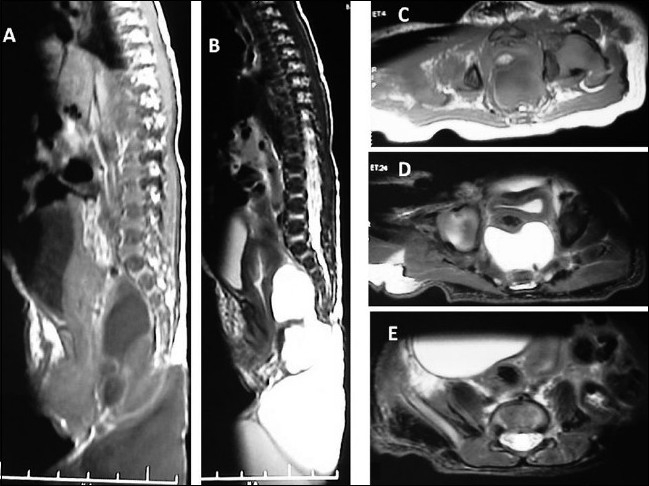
Sagittal T1 (A), T2 (B) and axial (C–E) magnetic resonance imaging revealed a multiloculated dumbell (both internal and external type) swelling between the sacrococcygeal spine and anorectal canal
